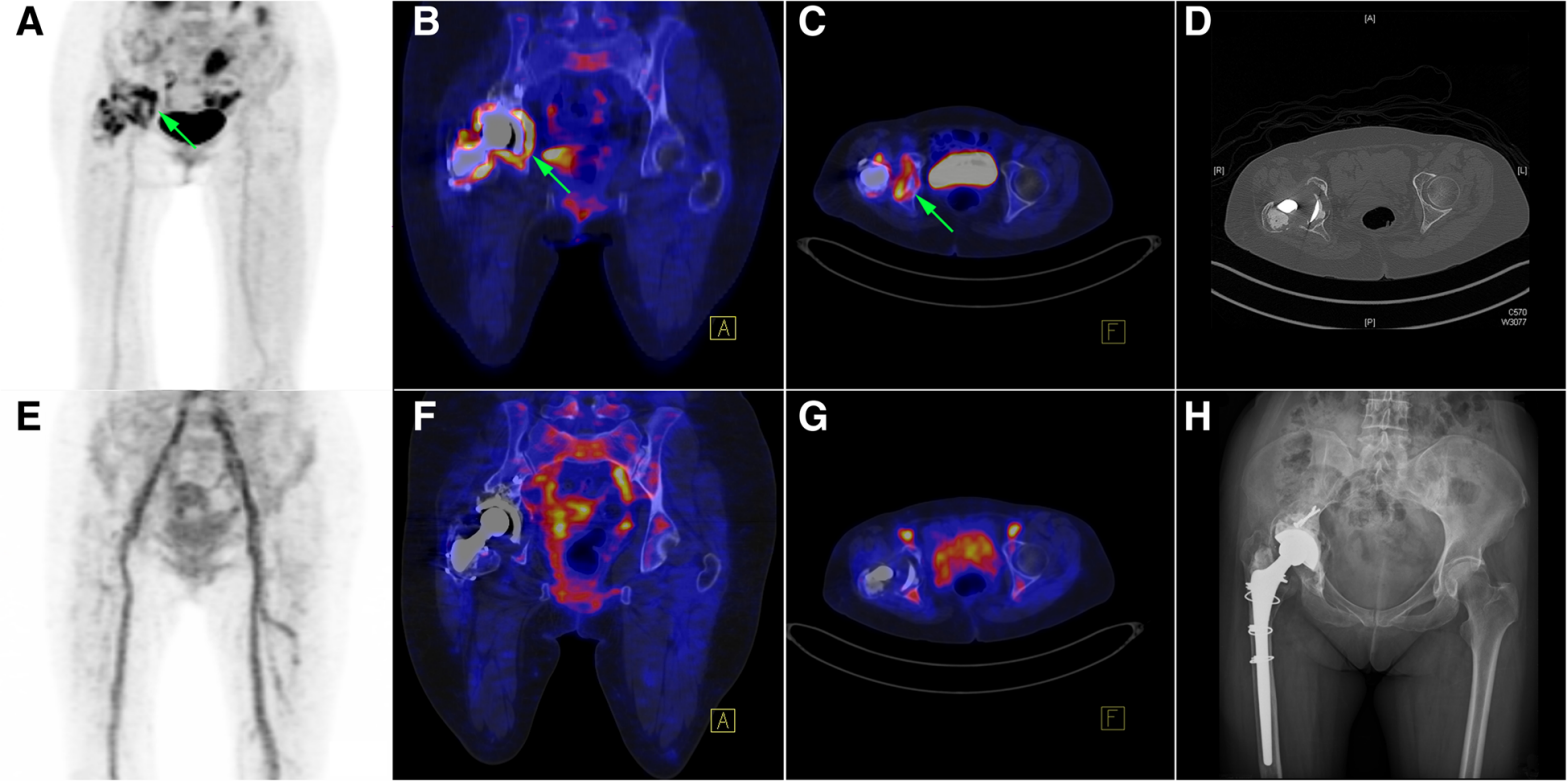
Patient (case # 16) with a history of right total hip arthroplasty performed 16 years before imaging. The results of ^18^F-FDG PET/CT (upper row) and ^68^Ga-citrate PET/CT (lower row) are presented. a–d Green arrows indicate an intense ^18^F-FDG uptake surrounding the cup and the proximal part of the hip prosthesis stem components. On CT images, osteolytic changes and the presence of residual cement in the right acetabulum were evident. Cup loosening with synovial hypertrophy and clear joint fluid were detected during surgery. ^18^F-FDG PET/CT findings were therefore classified as false positve. d–f Findings on ^68^Ga-citrate PET/CT were true negative, without any obvious radiotracer accumulation at the corresponding sites. h Plain film
